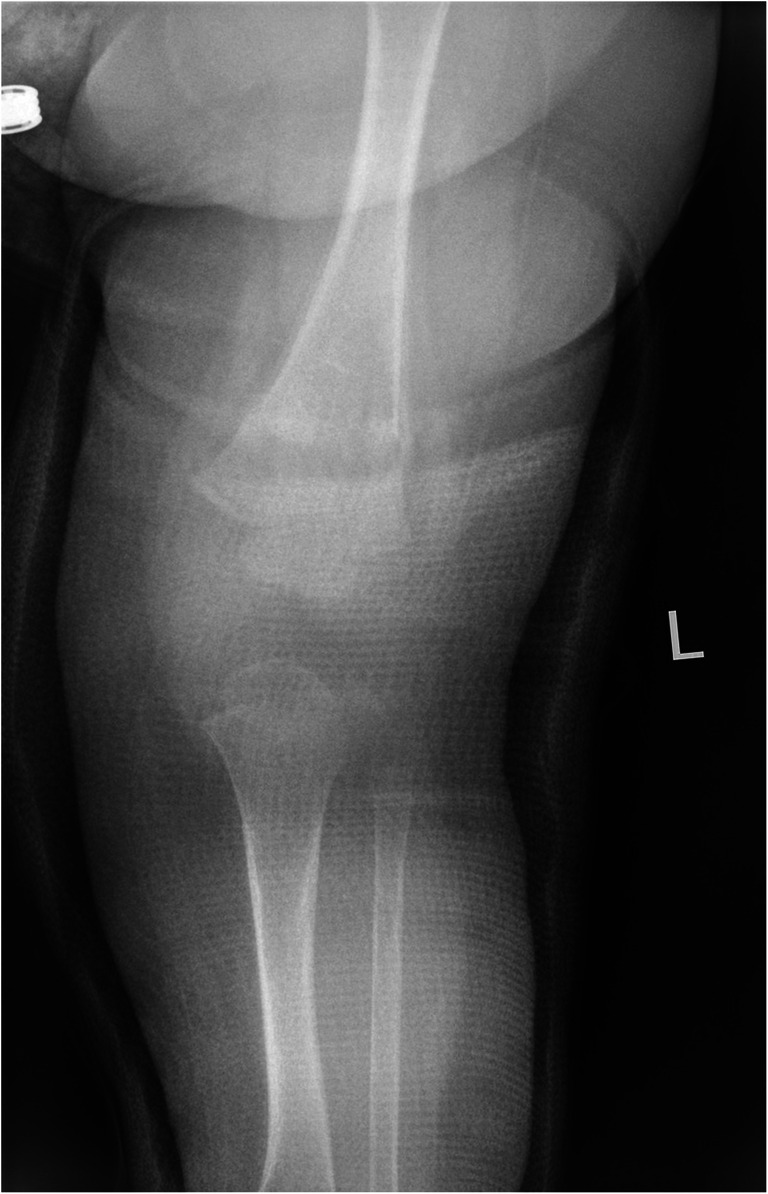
An anteroposterior radiograph of the left femur in an 8-month-old boy shows subperiosteal new bone formation
